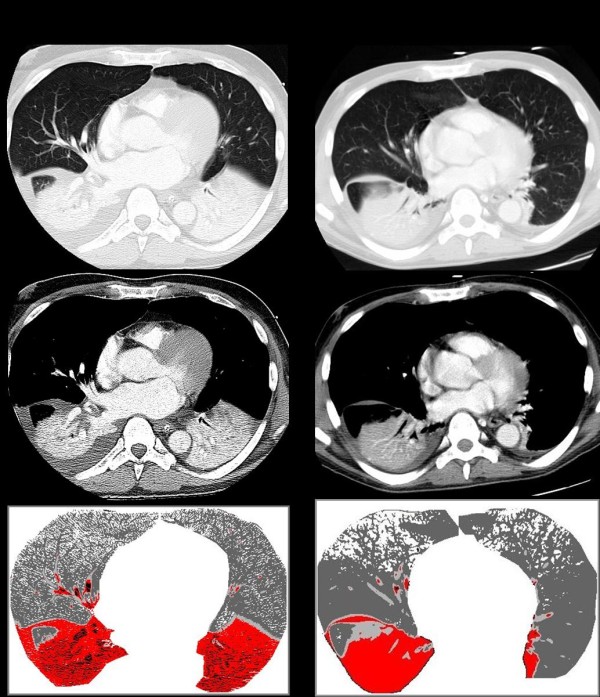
Chest CT performed on Day 3 and Day 37 after the onset of refractory hypoxaemia. a) Chest computed tomography (CT) on Day 3 following the onset of refractory hypoxaemia showing a bilateral consolidation of lower lobes with a right pleural effusion extending to the main fissura (upper CT section, parenchymal window). Large vessels are opacified within each lower lobe consolidation (middle CT section, mediastinal window). The application of a colour encoding system (lower part of the figure) confirms the consolidation of lower lobes [red colour, characterizing nonaerated lung regions with CT attenuations > -100 Hounsfield densities (HU)], shows the hypervascularization of lung consolidations (black colour, characterizing pulmonary vessels containing contrast material with CT attenuations > +200 HU) and evidences the hyperinflation of upper lobes (white colour, characterizing hyperinflated lung regions with CT attenuations < -900 HU). Normally (dark grey colour, characterizing lung regions with CT attenuations ranging between -900 and -500 HU) and poorly aerated lung regions (light grey colour, characterizing lung regions with CT attenuations ranging between -500 and -100 HU) are located to upper lobes. b) The same CT sections obtained at Day 20 following liver transplantation (37 days after the onset of refractory hypoxaemia) showing a persisting consolidation of the right lower lobe without pleural effusion. Large vessels are not anymore opacified within the consolidation (middle CT section, mediastinal window). The application of the colour encoding system (lower part of the figure) confirms the consolidation, the presence of hyperinflated lung regions in upper lobes. It does not show anymore hypervascularization within the right consolidation, suggesting a regression of HPS.
